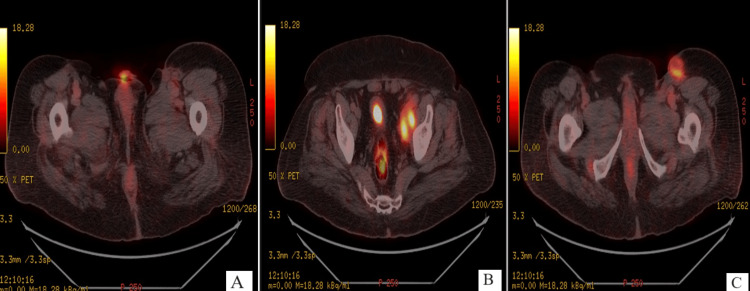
PET CT scan with a hypermetabolic focus in the stump of the penis (A, SUVmax of 7.5) in favor of local recurrence, with extensive pathological active lymph node involvement in left pelvic lymph node (B, SUVmax of 7.9) and in the left inguinal lymph nodes (C, SUVmax of 6.4) SUVmax: standardized uptake value
